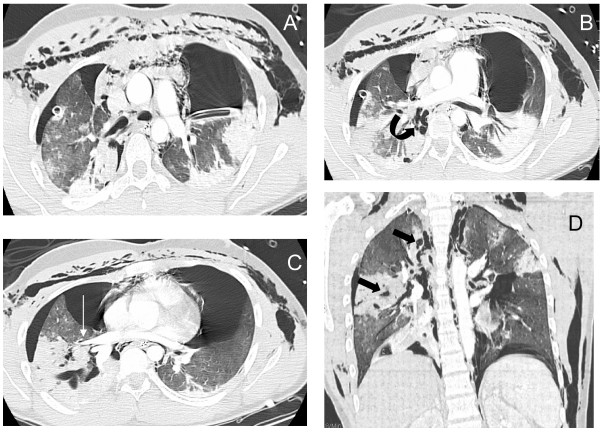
Computed tomography scan following emergency chest tube drainage. Axial 1.25 mm thick sections with a lung window. (a) Persistent bilateral pneumothorax, pneumomediastinum and extensive subcutaneous emphysema. (b) Multiple lucencies around the right bronchial tree (curved arrow) precluding the correct recognition of the bronchial rupture. (c) The Macklin effect around the right lower pulmonary vein (white arrow). (d) Coronal view demonstrating multiple areas of alveolar consolidation in the right upper and lower lobes: intraparenchymal lucencies resulting from lung lacerations are visible on the right side (thick arrows).
